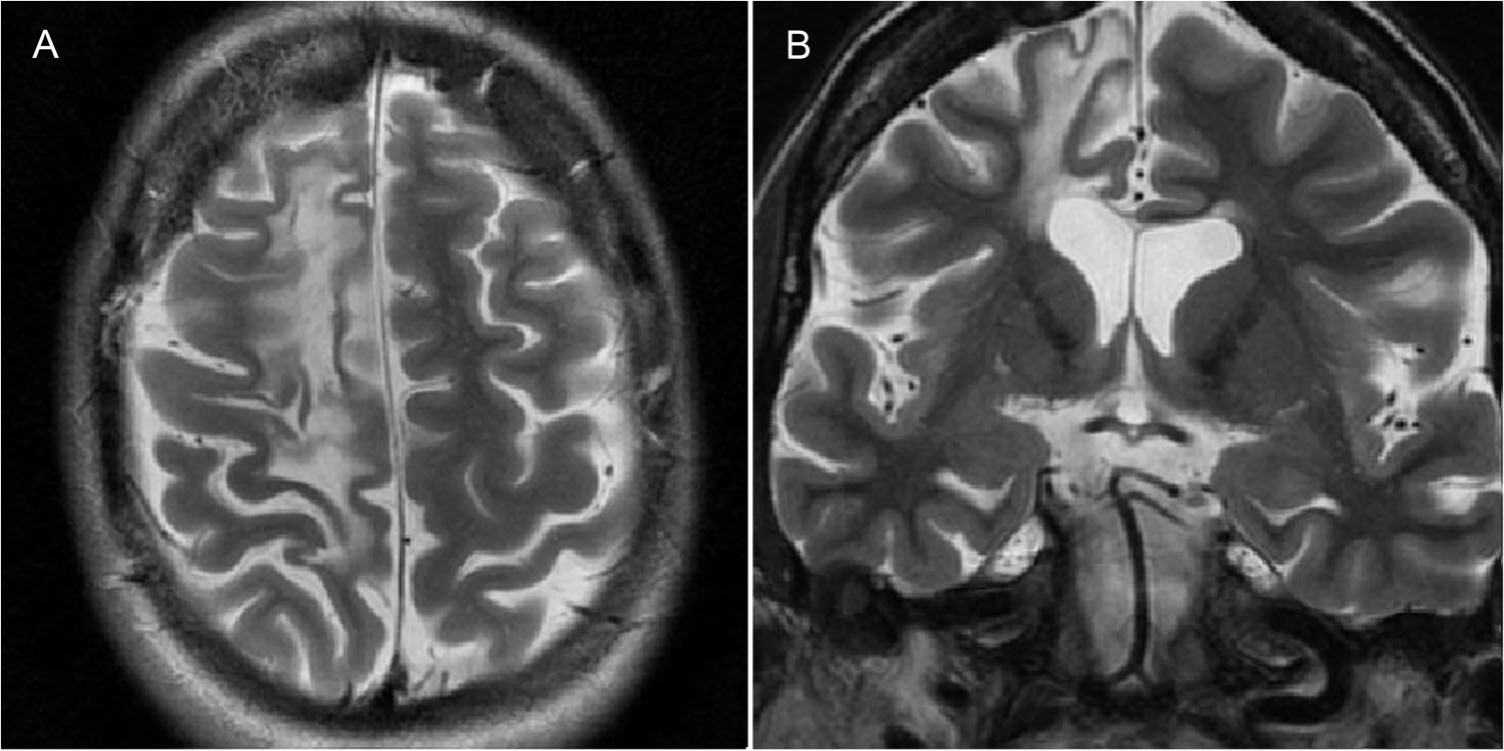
Rasmussen encephalitis confirmed via biopsy in a 37-year-old woman with refractory partial motor epilepsy. A) An axial T2-weighted image and B) a coronal T2-weighted image showing atrophy and gliosis of the right frontal lobe.
